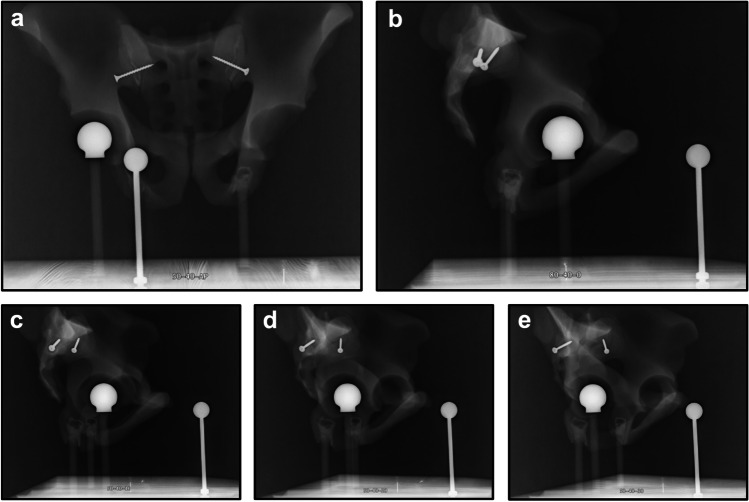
Depiction of a series of one a.p. radiograph with four corresponding lateral radiographs. In this example, the ECM is positioned at 50 mm anterior offset and 40 mm lateral offset. a a.p. radiograph and b lateral radiograph without rotation. c Lateral radiograph with 10° rotation. d Lateral radiograph with 20° rotation. e Lateral radiograph with 30° rotation
